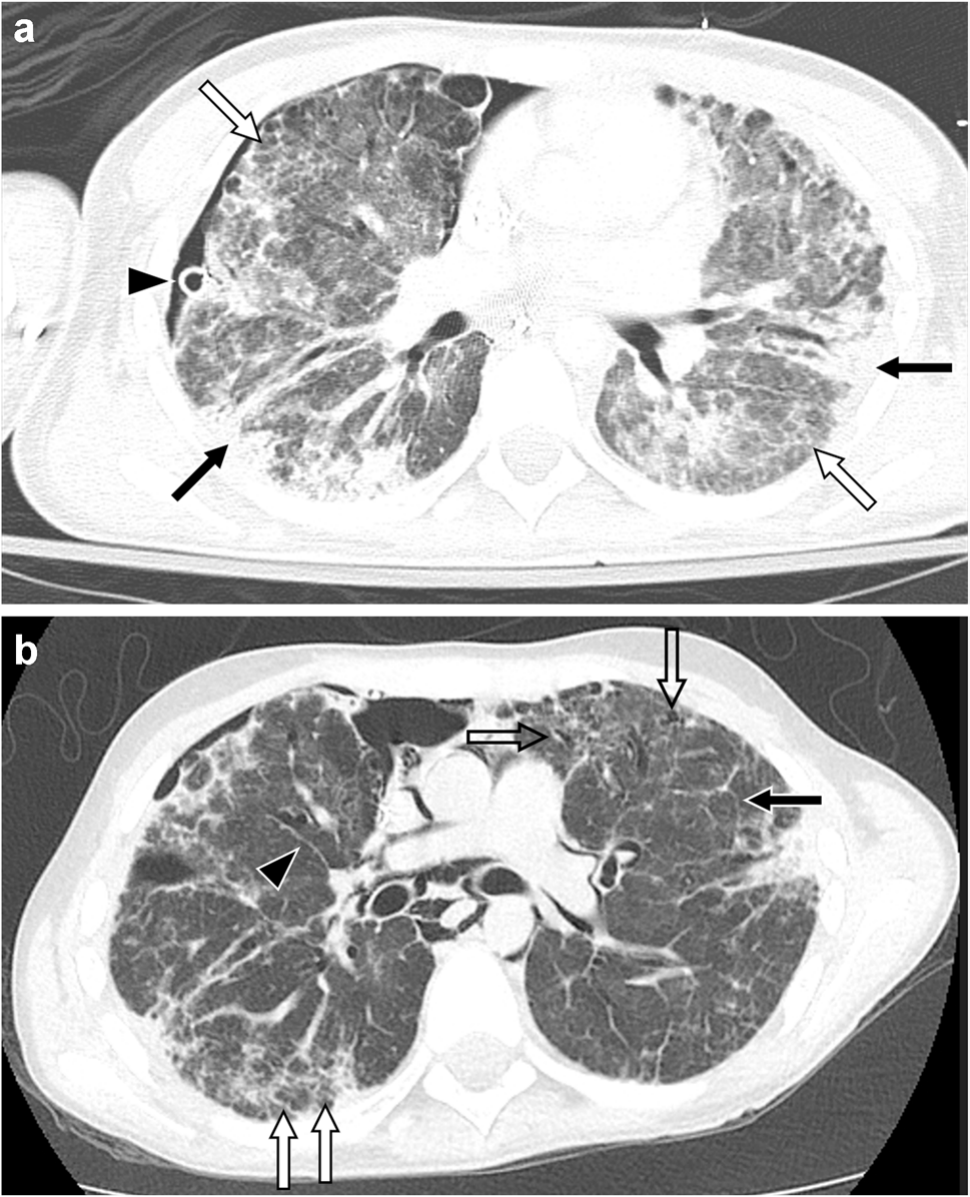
A 14-year-old receiving trimethoprim-sulfamethoxazole for acne who developed acute respiratory distress syndrome related to acute eosinophilic pneumonia. a Axial CT image without contrast showing diffuse bilateral ground-glass opacity, multifocal bilateral consolidation (*black arrows*) reticulation (*white arrows*), and a small right pneumothorax with chest tube in place (*arrowhead*). b Axial image from follow-up CT without contrast obtained 7 weeks later after interval corticosteroid treatment and discontinuation of the trimethoprim-sulfamethoxazole showing decreased ground-glass opacity and consolidation, consistent with resolving medication-induced lung disease. Interlobular septal thickening (*black arrow*), reticulation (*white arrows*), and band-like opacities (*arrowhead*) associated with bronchiolectasis (*open arrows*) remain suggesting residual fibrosis
